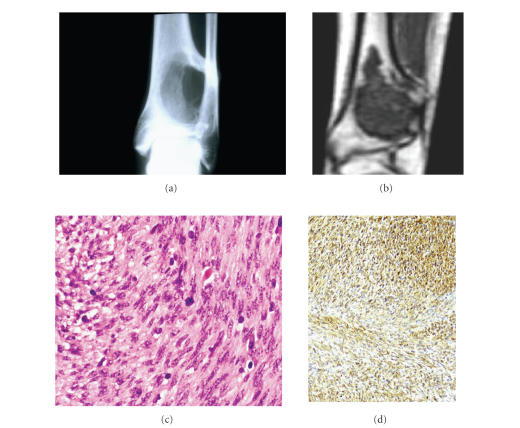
(a) Conventional radiograph reveals an osteolytic lesion in metadiaphysis of the left distal tibia with cortical destruction. (b) T1-Weightened Magnetic Resonance Image shows a well-defined low density intramedullary location with cortical breakthrough. (c) Interlacing
